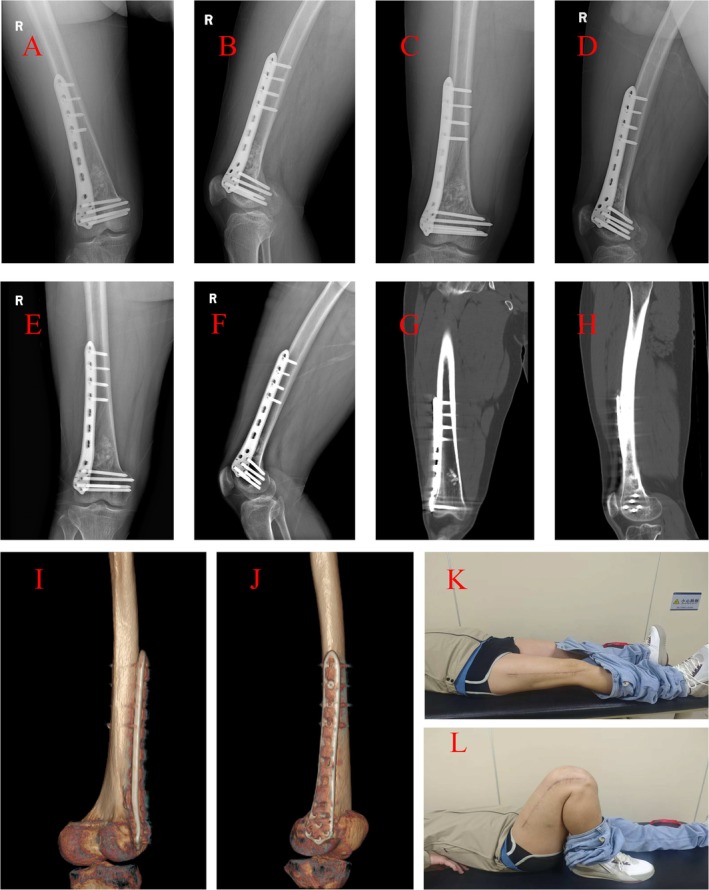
Postoperative follow‐up and functional recovery. (A,B) Radiographs at 1 month. (C,D) Radiographs at 3 months showing early callus formation without fixation loosening. (E–J) Radiographs and computed tomography images at 6 months confirming complete union and integration of the autologous bone graft. (K,L) Clinical photographs showing knee range of motion from 10° to 140°.
